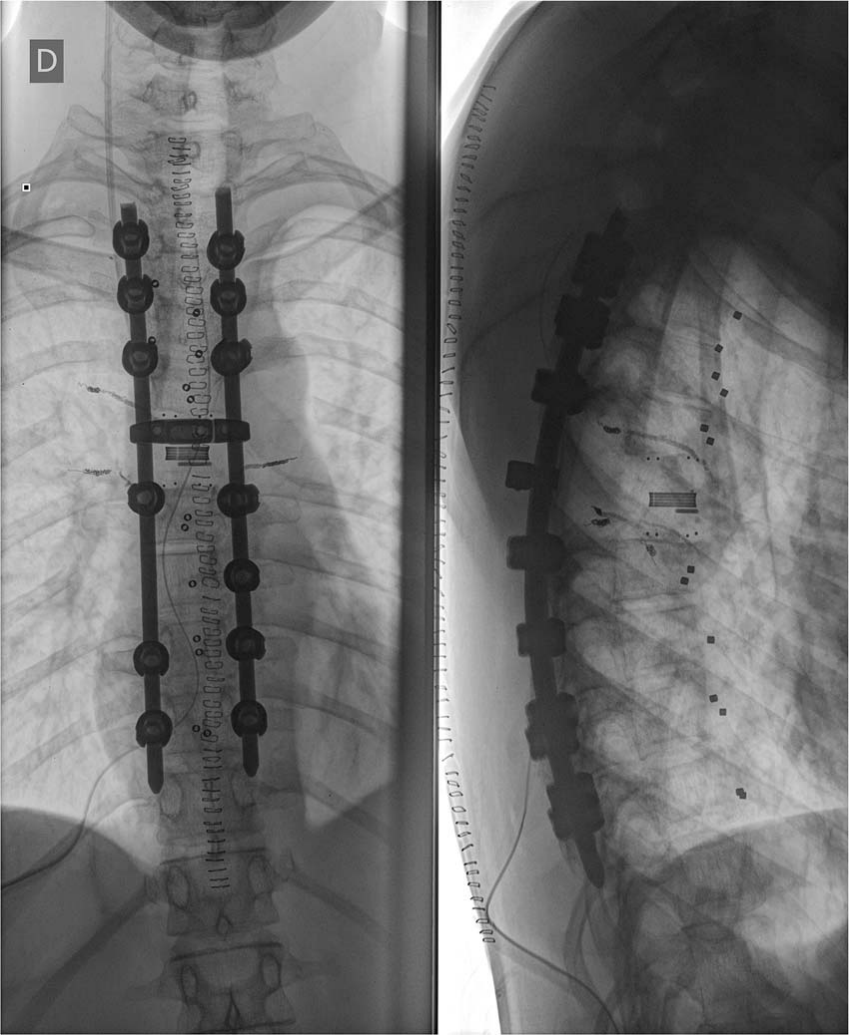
Postoperative X-ray imaging immediately after surgery; anteroposterior view (left) and lateral view (right).
